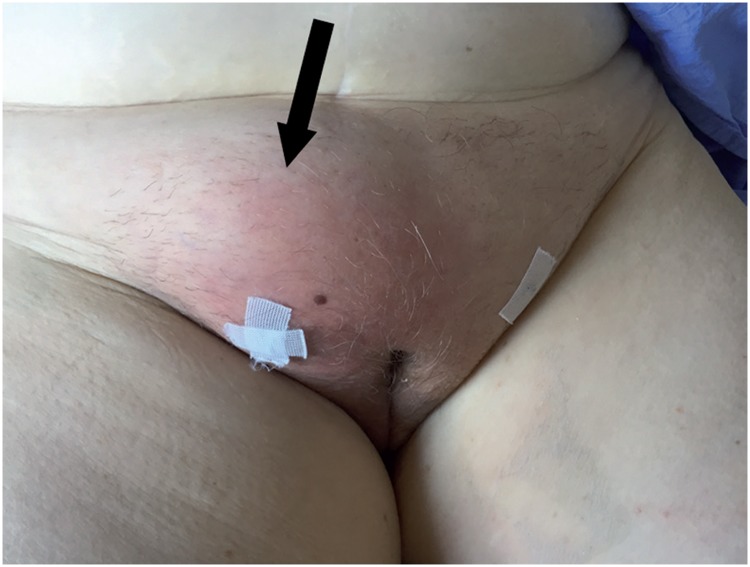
Large subcutaneous abscess (arrow) after transobturatory SSS treated with ultrasound guided puncture.
